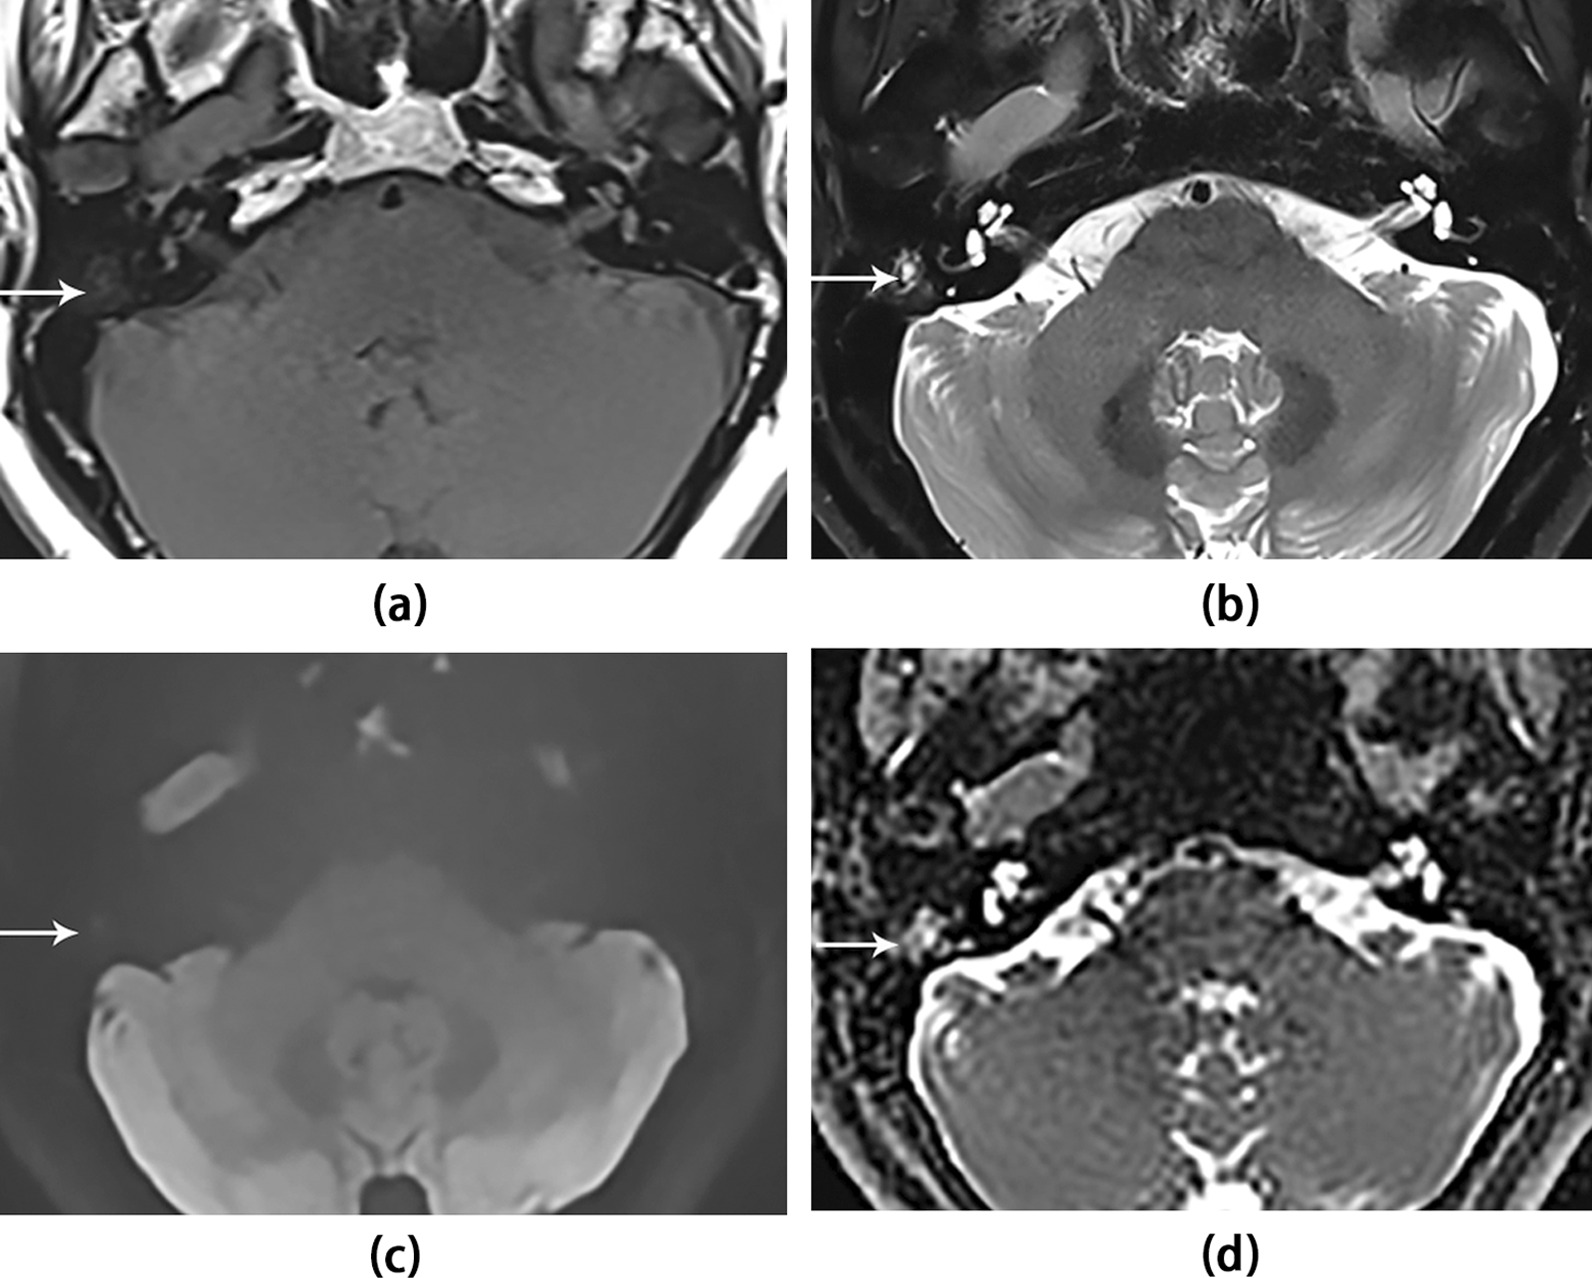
A 41-year-old female patient with true-negative NCS on the right side. Axial images show the lesion (white arrow) as low signal on (a) the T1WI and (c) TGSE BLADE DWI b1000 image, and high intensity on (b) the T2WI-FS and (d) ADC map
